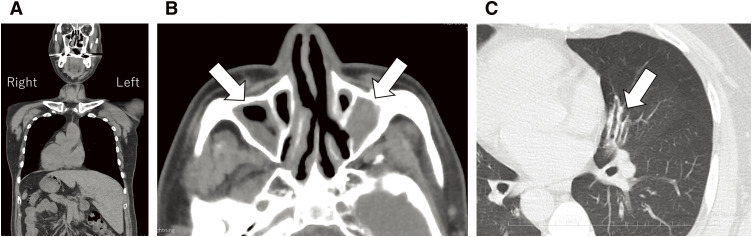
CT demonstrated complete visceral inversion (situs inversus totalis) (A). Mucosal thickening and fluid collection were observed in the sinuses (B). Ectasias in several bronchus was also observed (C).
